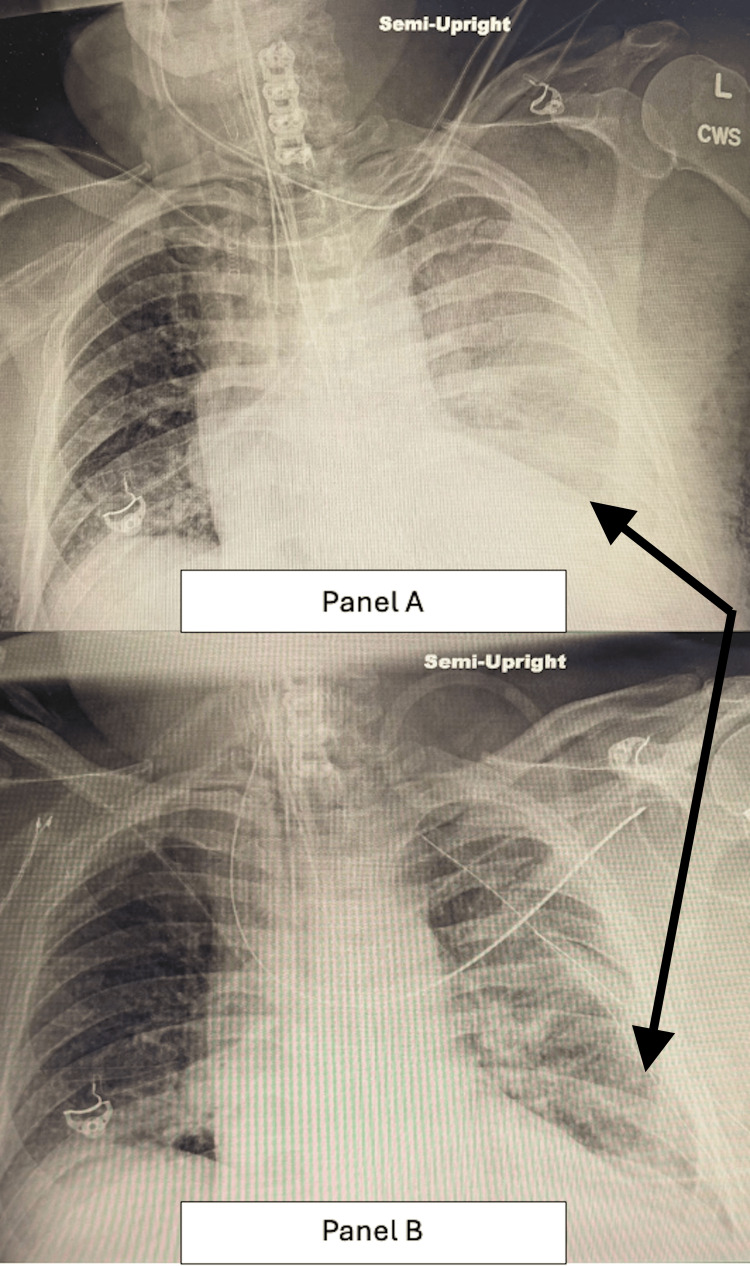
Chest X-rays before and after chest tube placement showing improvement with residual left pleural effusion. (A) Chest X-ray prior to chest tube placement with bilateral infiltrates (diffuse on the left and involving the lung base on the right) and left pleural fluid (black arrow). (B) Chest X-ray one day status post chest tube placement, improved from previous, with stable bilateral infiltrates and small residual left pleural effusion (black arrow). CWS: cervical spine view.
